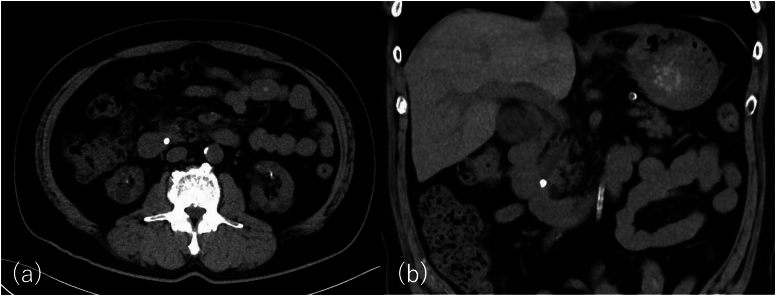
Abdominal computed tomography at the time of hospitalization. Abdominal computed tomography showing common bile duct dilation and common bile duct stone in axial (A) and coronal (B) views.
